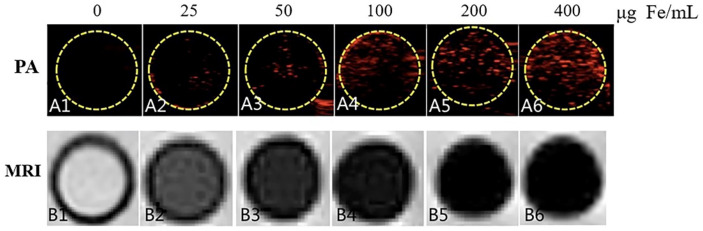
Dual-modal PA and MRI imaging ability of magnetic PLGA-IO MPs. Qualitative observations show an enhanced PA signal with increasing iron content in the particles and a low signal on MRI [84]. Copyright 2018, Public Library of Science.
